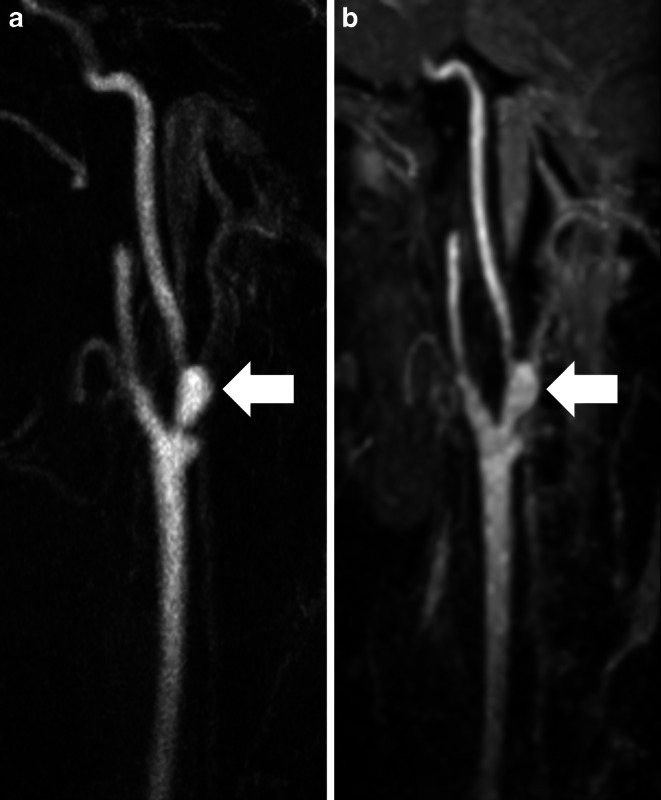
Maximum intensity projections of the right cervical arteries in sagittal plane (slice thickness: 30 mm) in a 41-year-old male patient presenting acute onset of paresthesia in the left hand. Pseudoaneurysm of the proximal extracranial internal carotid artery (arrow) with about 8 mm diameter is shown as additional finding in contrast-enhanced MRA (a) and Relaxation-Enhanced Angiography without Contrast and Triggering (REACT, water-only; (b)). Diagnosis was confirmed using duplex ultrasound. REACT provides better vessel delineation with less image noise
